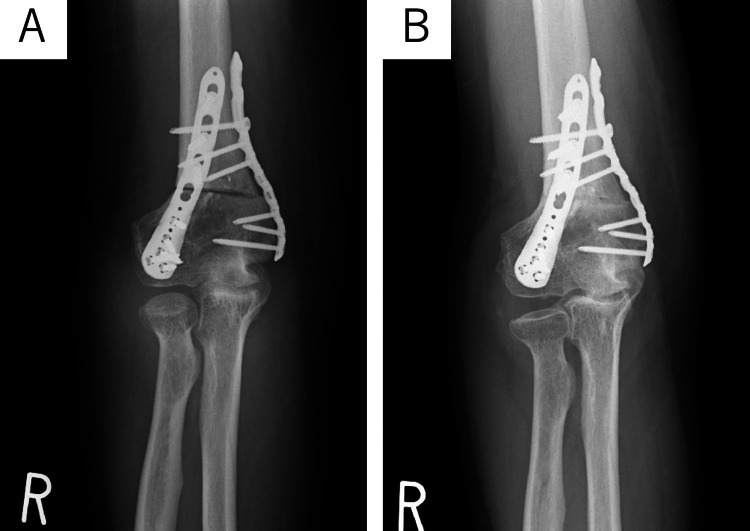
Postoperative radiograph of case two (A) Postoperative radiographs confirmed a corrected carrying angle (CA) of 5 degrees. (B) The osteotomy site had achieved bone union and CA was preserved at six months.
